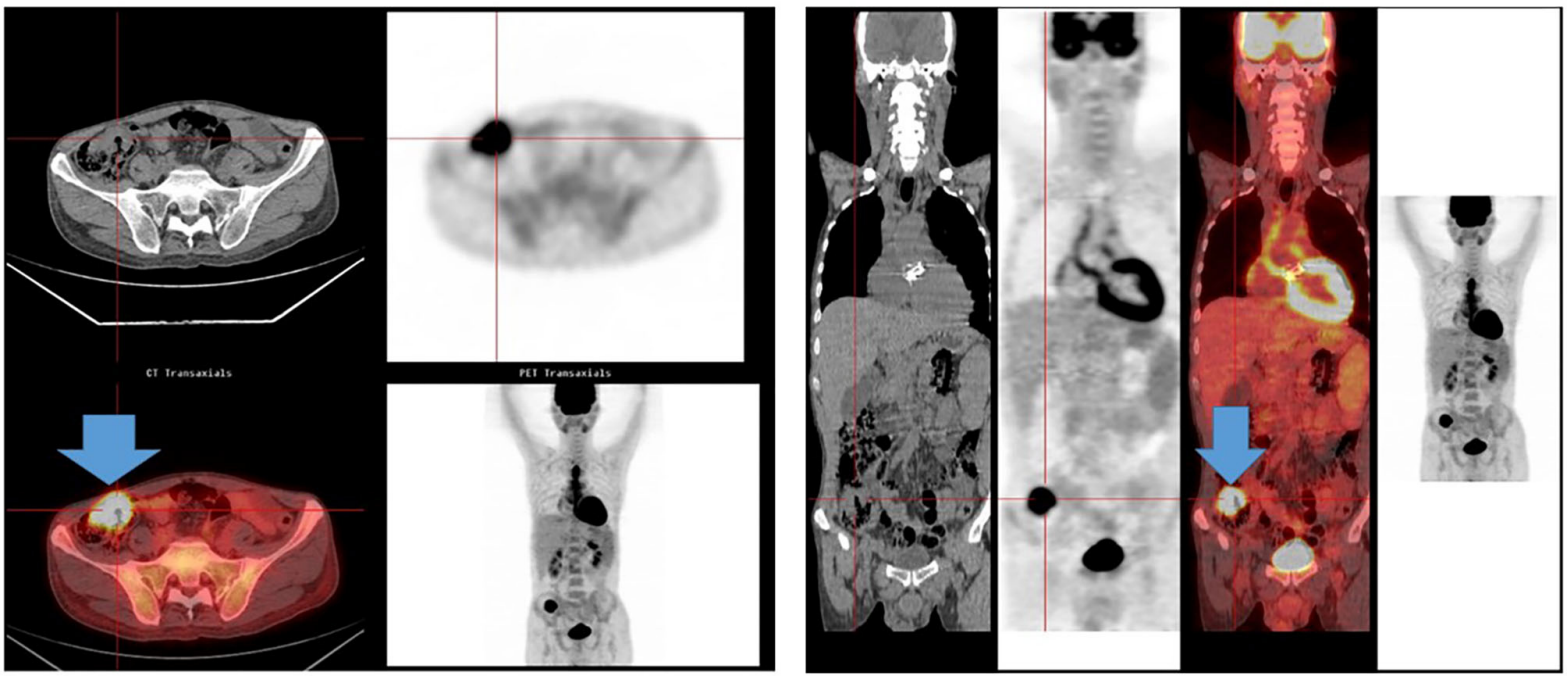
PET/CT and colorectal cancer. A 60-year-old man was admitted for *Streptococcus gallolyticus*; the PET/CT showed an intense hypermetabolic focus in the cecum, suggesting a colorectal cancer. A colorectal adenocarcinoma was diagnosed after colonoscopy.
